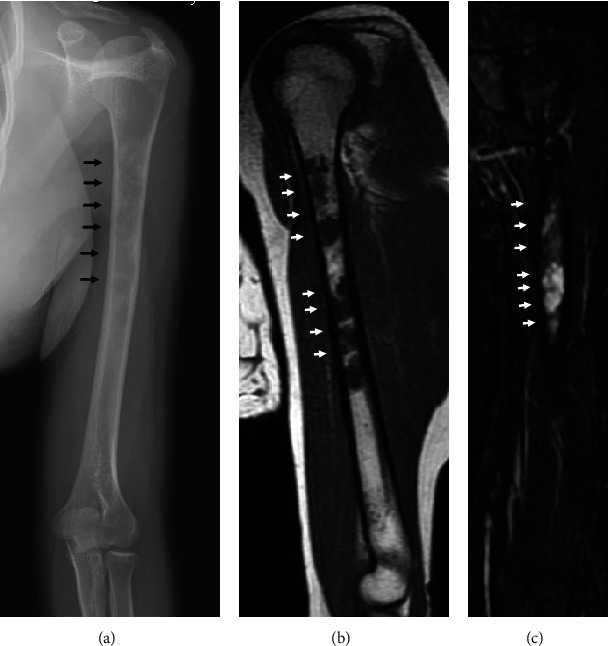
Plain radiograph and magnetic resonance imaging. (a) Plain radiograph showing radiolucent region and no periosteal reaction on the left proximal humerus (arrows). (b) Plain magnetic resonance imaging showing a low-signal-intensity region on T1-weighted scan. (c) High-signal-intensity lesion on short-tau inversion recovery.
